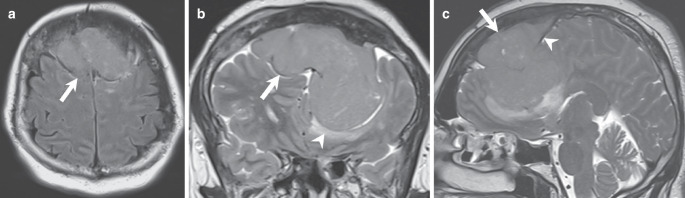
Axial (a) fluid attenuated inversion recovery (FLAIR) images, as well as coronal (b), and sagittal (c) T2 weighted images confirmed the presence of an extra-axially located bifrontal lesion displaying homogeneous isointensity relative to the cortex (a–c, arrow). Perifocal edema was observed along the margins of the basal aspects of the lesion (b, arrowhead). Portions of the lesion (c, arrow) were clearly situated underneath the dura mater (c, arrowhead)
